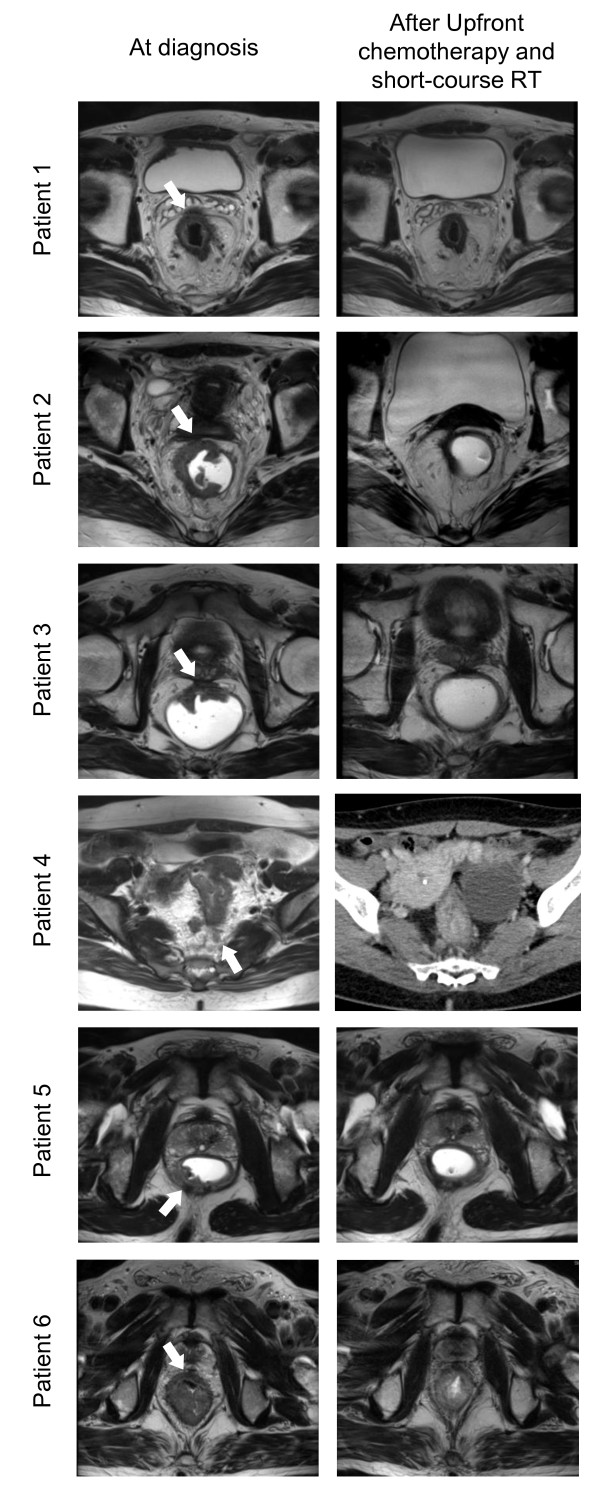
MRI or CT at diagnosis and after upfront chemotherapy and short-course RT for each patient. Arrow points to the mesorectal fascia involvement or closeness. After preoperative treatment, regression of rectal mass or lymph node was observed.
